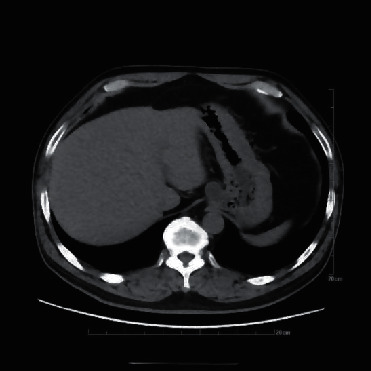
CT scan revealed a lesion at the gastric cardia with slightly low density, it was with a cystic wall-like structure, protruding outward, and adjacent vessels were found.
